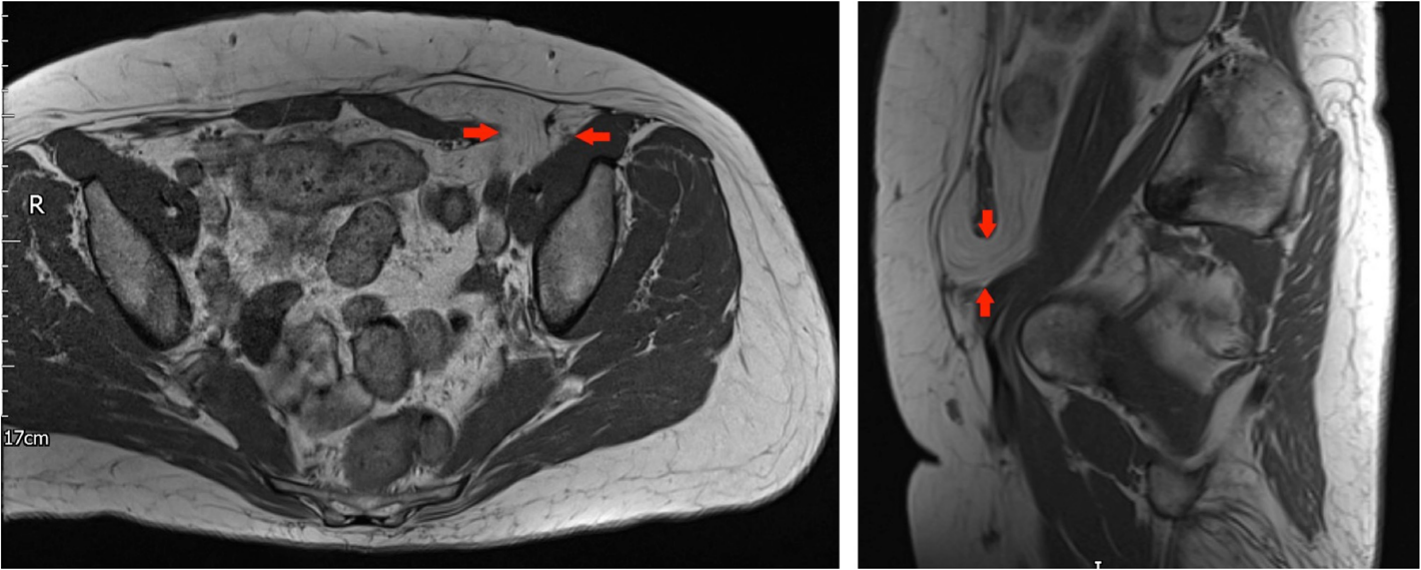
Left panel: Axial T1-weighted MRI of the pelvis and lower abdomen: red arrows outline a defect in the linea semilunaris with a fat containing hernial sac. Right panel: Sagittal T1-weighted MRI of the pelvis and lower abdomen: Spigelian hernia outlined by red arrows with hernial sac extending to the level of the pubic symphysis
